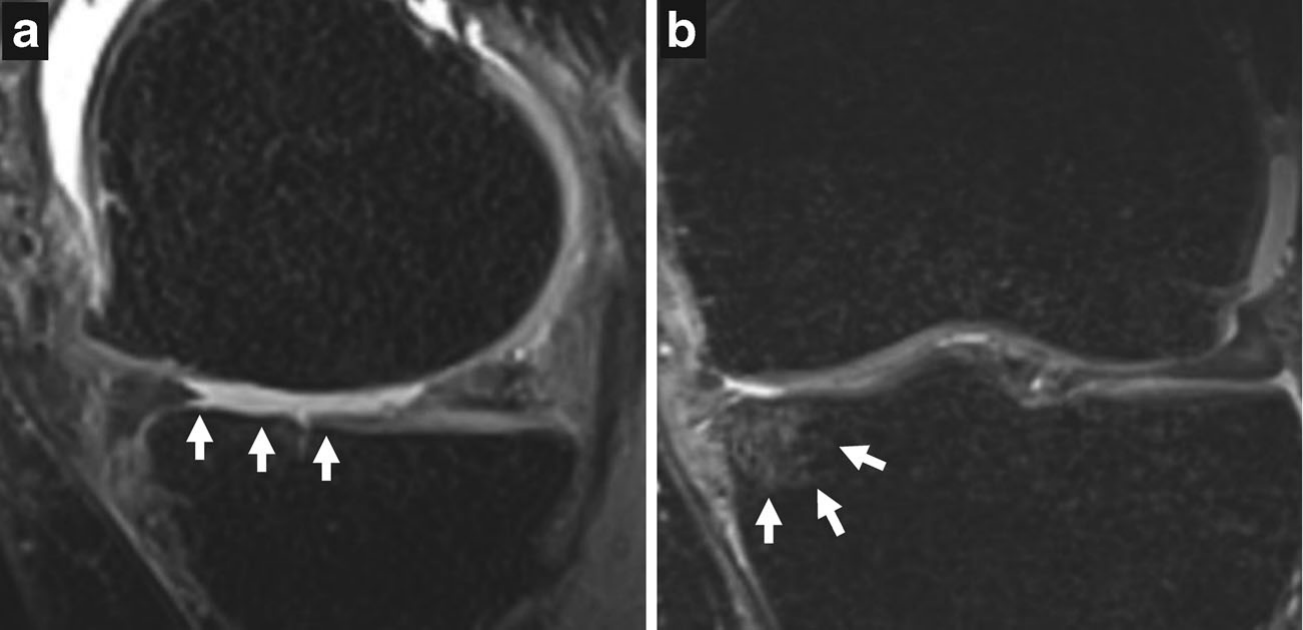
Example MR images obtained using proton density-weighted SPACE fat-suppressed turbo spin-echo sequence. A) Grade 3 for the size of cartilage loss in anterior and grade 2 cartilage loss in central medial tibia (*arrows*). B) Grade 2 for the size of bone marrow lesion in medial central tibia (*arrows*)
